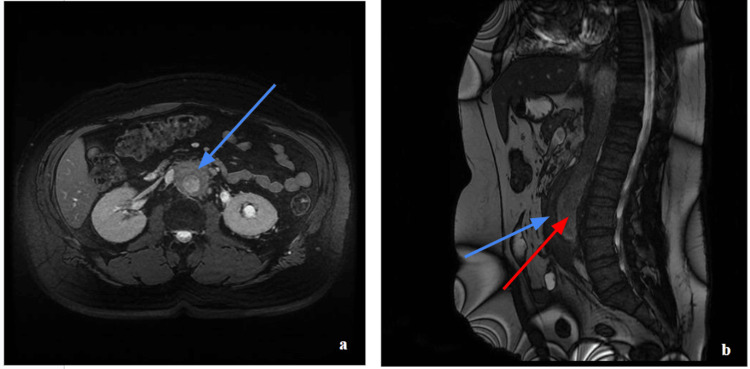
Abdominal MRI demonstrating retroperitoneal fibrosis with associated distal abdominal aortic aneurysm. Axial (a) and sagittal (b) abdominal MRI images demonstrating confluent retroperitoneal soft-tissue thickening surrounding the abdominal aorta and ureters (blue arrows), in keeping with retroperitoneal fibrosis. A fusiform aneurysm of the distal abdominal aorta is also noted (red arrow).
